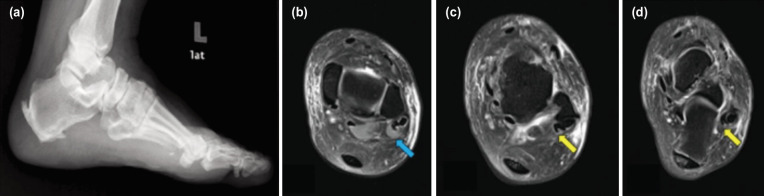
(a) Presence of calcaneal spur on lateral view of left foot and ankle radiograph. (b) The blue arrow showing ankle joint’s MRI of the peritendinous fluid within the peroneal tendon sheath. (c, d) The yellow arrows showing ankle joint’s MRI of longitudinal tear of either peroneal tendons.
